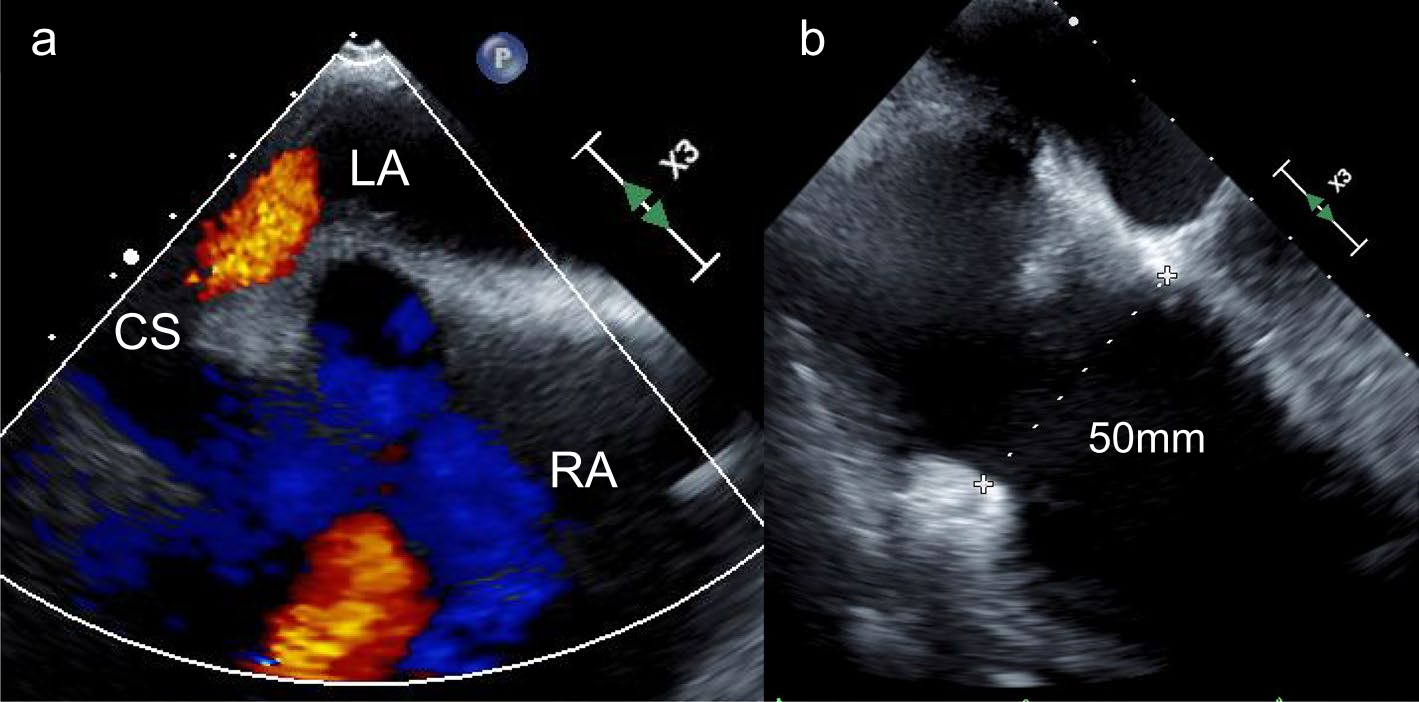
Transesophageal echocardiography revealed a direct communication between the left atrium and CS with left-to-right shunt flow, as observed via color Doppler imaging (a). The annulus of tricuspid valve was dilated at end-diastole, 50 mm in diameter (b). LA, left atrium; RA, right atrium; CS, coronary sinus
